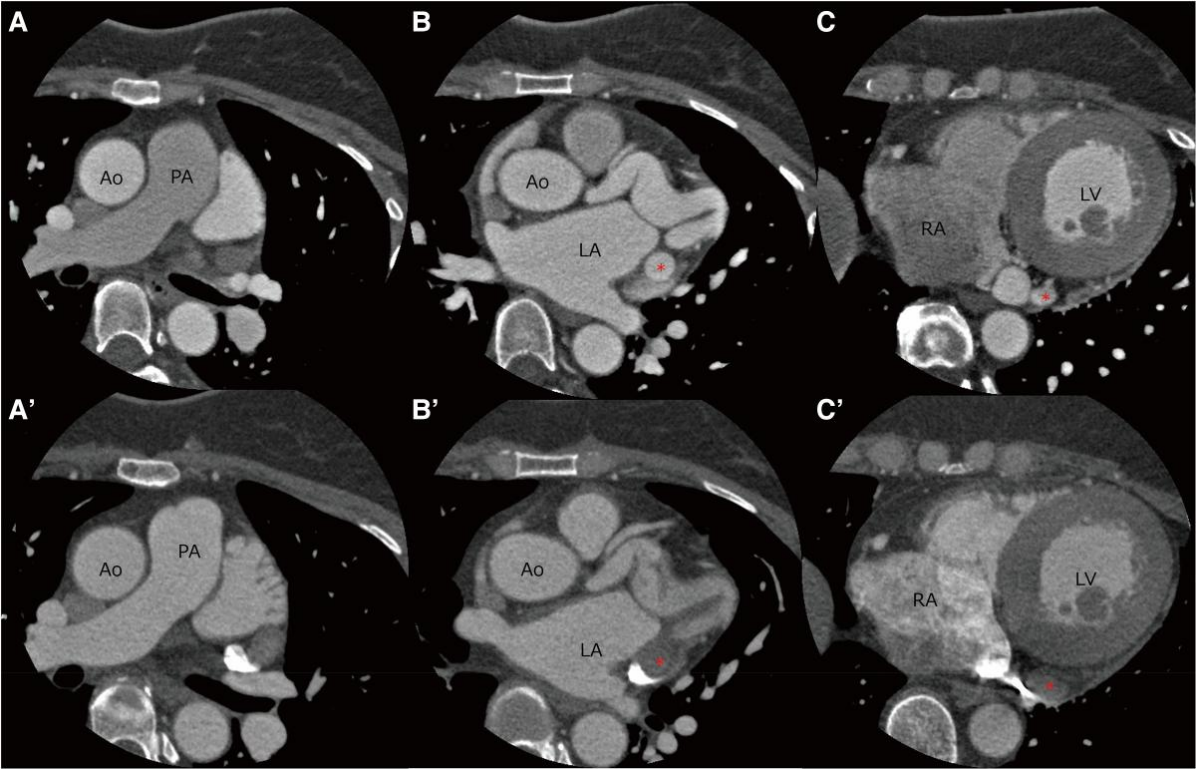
contrast-enhanced computed tomography before and after 6 months of embolization. Contrast-enhanced computed tomography before (*A–C*) and after coil embolization (*A′–C′*). Follow-up contrast-enhanced computed tomography images show the absence of contrast opacification in the previously visualized fistulous vessel (red asterisk), indicating successful occlusion. Ao, aorta; LA, left atrium; LV, left ventricular; PA, pulmonary artery; RA, right atrium.
